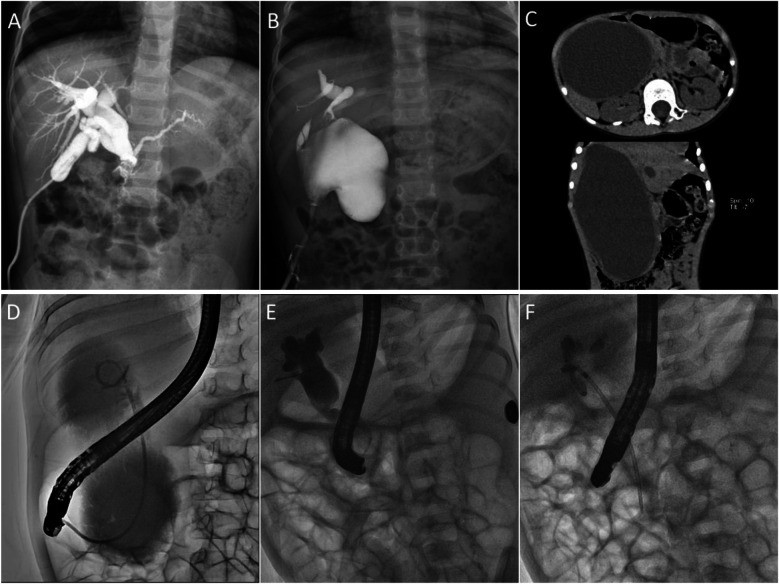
Imaging findings of different drainage modalities. (A) Laparoscopic external drainage of the gallbladder; (B) Laparoscopic external drainage of the bile duct; (C,D) A 5-year and 7-month-old child with a substantial common bile duct associated with severe cholangitis underwent EBD. (C) CT image indicated that the cysts were huge, and the size of the cysts was about 16 × 10 cm. (D) Under fluoroscopic guidance during ERCP, a 7-French nasobiliary catheter was selectively positioned within the cyst to establish continuous biliary decompression, and the cysts shrunk to 4 × 3 cm three weeks after the operation. (E,F) Under fluoroscopic ERCP guidance, a 7 Fr–7 cm pancreatic duct stent was placed reversely for internal biliary drainage.
